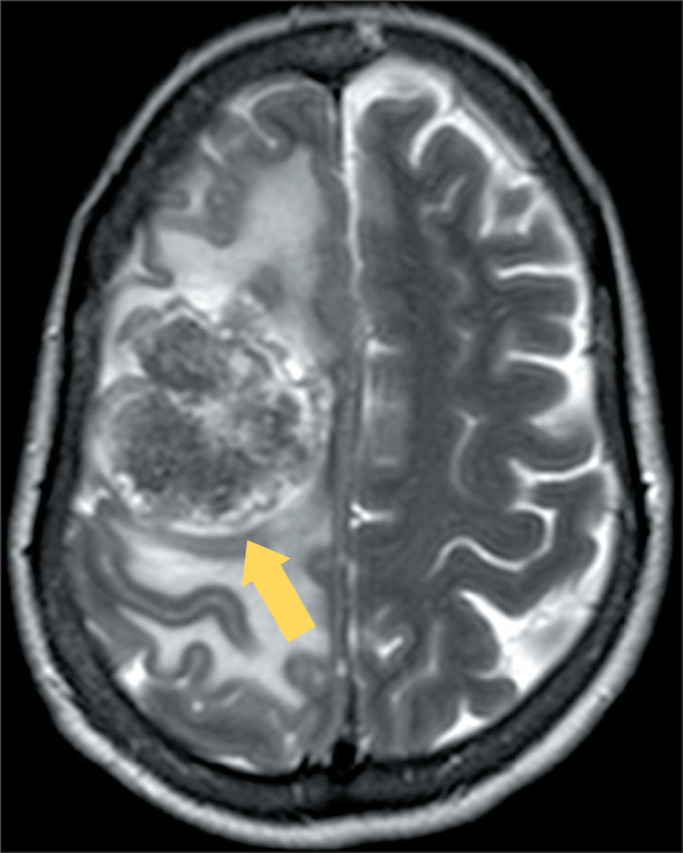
MRI of a patient with metastasis from a pulmonary neuroendocrine tumor.
